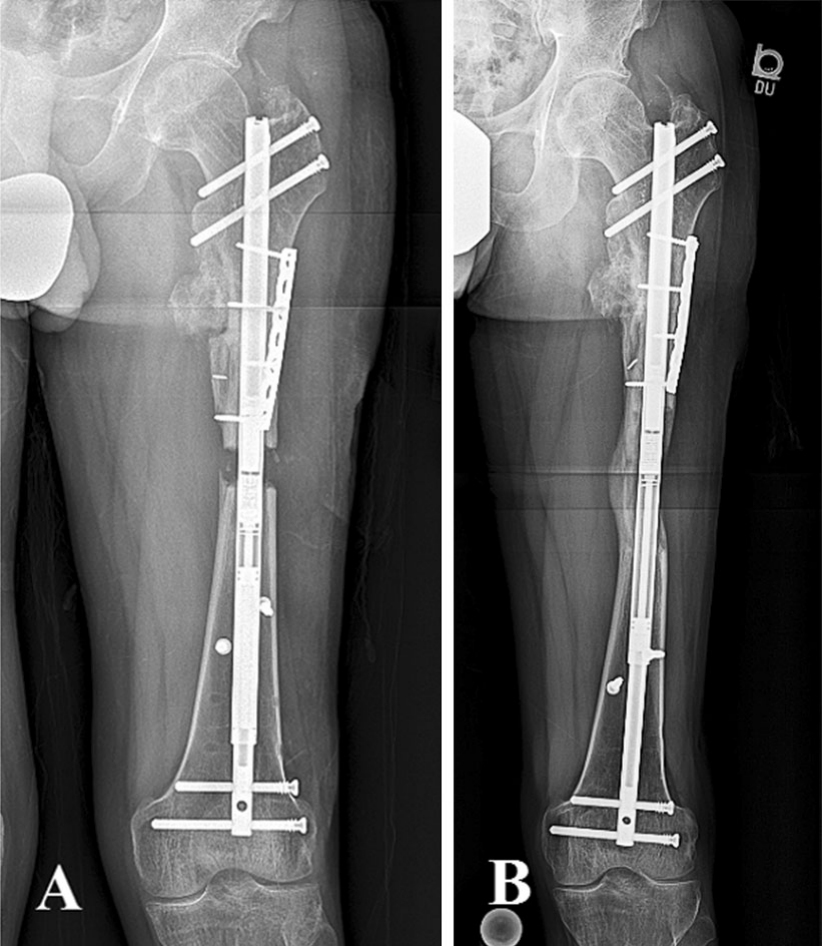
Antegrade femoral nail. a, b Represent radiographs taken at early distraction and consolidation phases, respectively. Blocking screws were not needed in the proximal fragment. One medial screw and one lateral screw were inserted in the distal fragment near the osteotomy site to prevent varus or valgus tilt of the fragment
